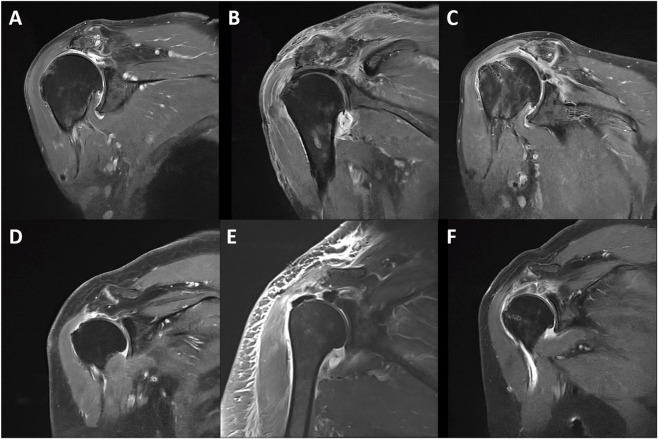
Representative MRI images preoperatively, at the day after surgery, and at 6 months postoperatively. (A–C) from one patient at different timepoints: (A) before surgery; (B) the day after surgery; (C) at 6 months follow-up. (D–F) from another patient at different timepoints: (D) before surgery; (E) the day after surgery; (F) at 6 months follow-up.
